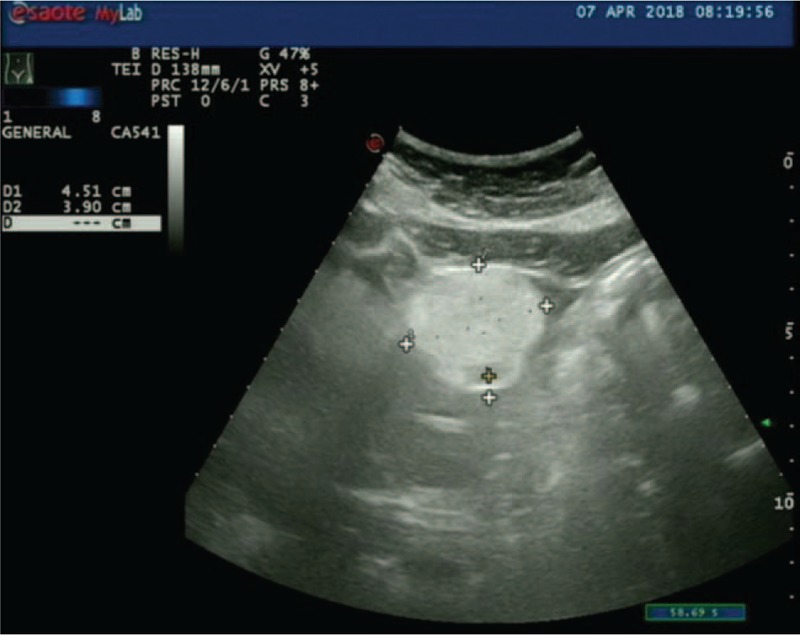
The B-type ultrasonic inspection showed that a hyperechoic nodule can be seen in hepatic left lateral, about 4.5×3.9 cm^2^ in size, with a clear boundary, which is considered a hepatic hemangioma.
